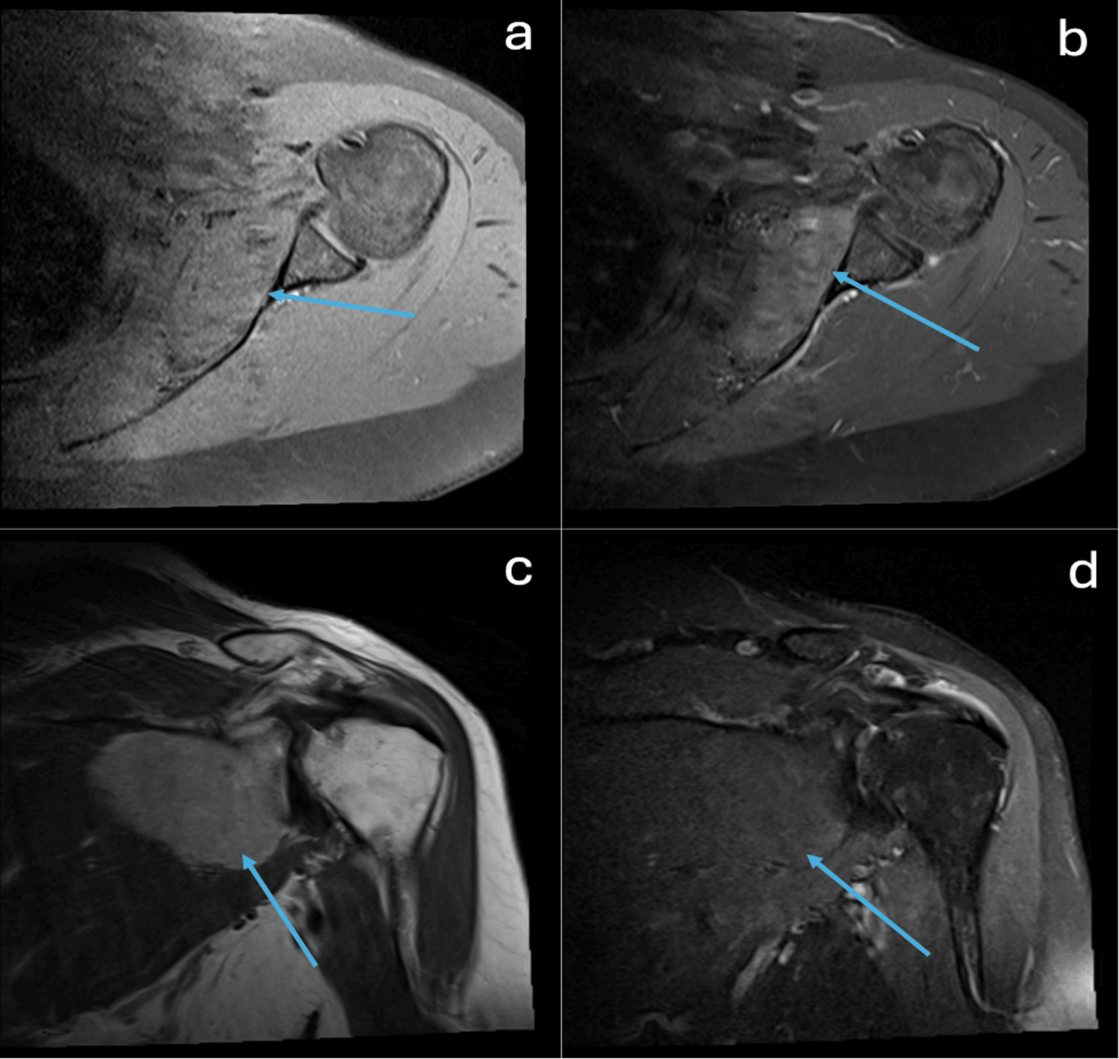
(a) Axial T1-weighted fat-suppressed MRI of the left shoulder pre-gadobutrol demonstrating a lobular 5.8 x 2.4 × 5.4 cm T1 heterogeneous mass. Notably, the mass did not suppress on the fat-suppression images. (b) Axial T1-weighted fat-suppressed MRI of the left shoulder shows patchy enhancement of the mass post-gadobutrol. (c) Coronal T2-weighted MRI of the left shoulder demonstrates that the lesion is hyperintense to skeletal muscle and mildly hypointense to subcutaneous fat. (d) Coronal T2-weighted fat-suppressed MRI of the left shoulder shows incomplete fat suppression of the mass.
